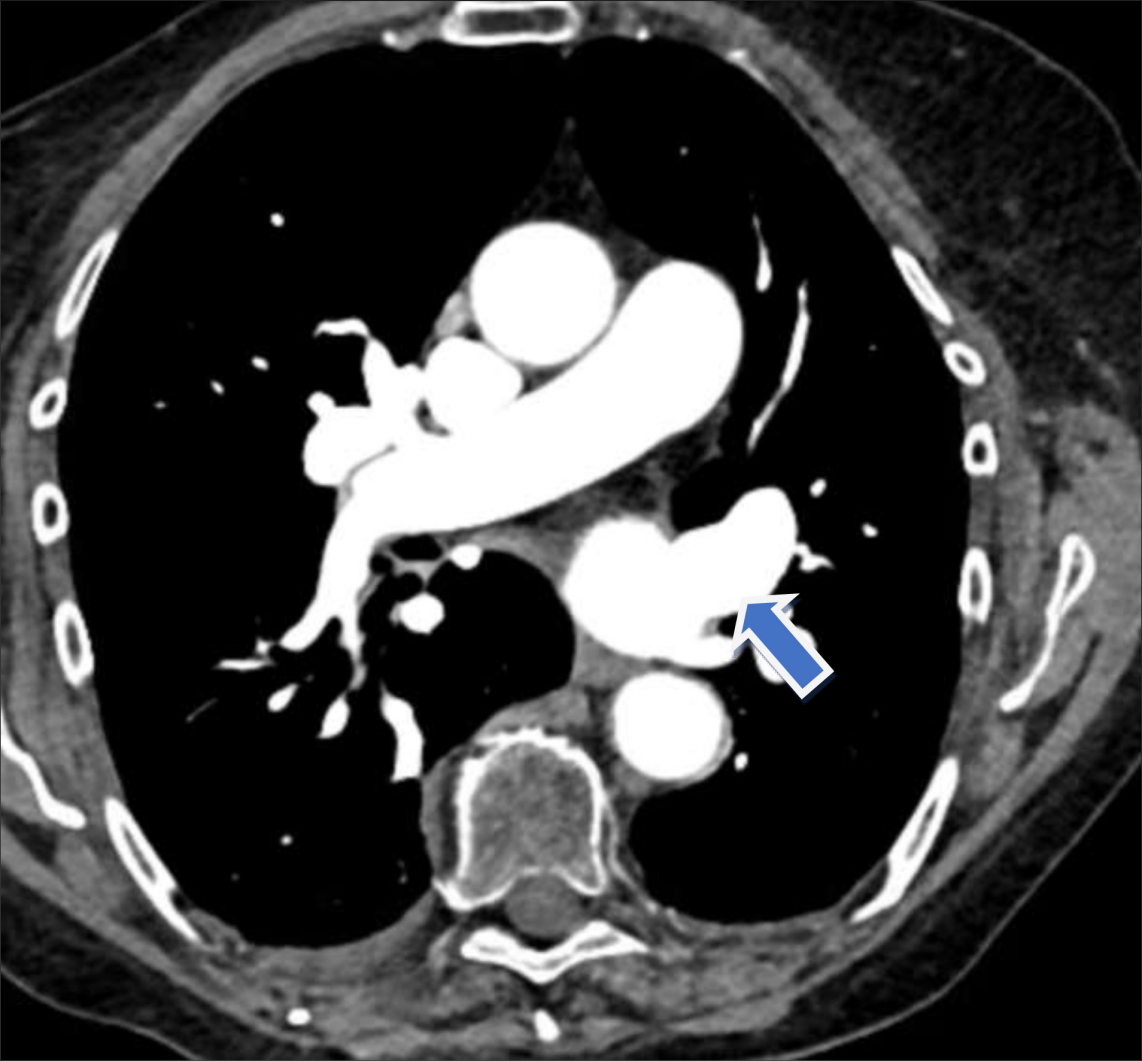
Computed tomography of the chest showing no thrombus in the left upper pulmonary vein.
